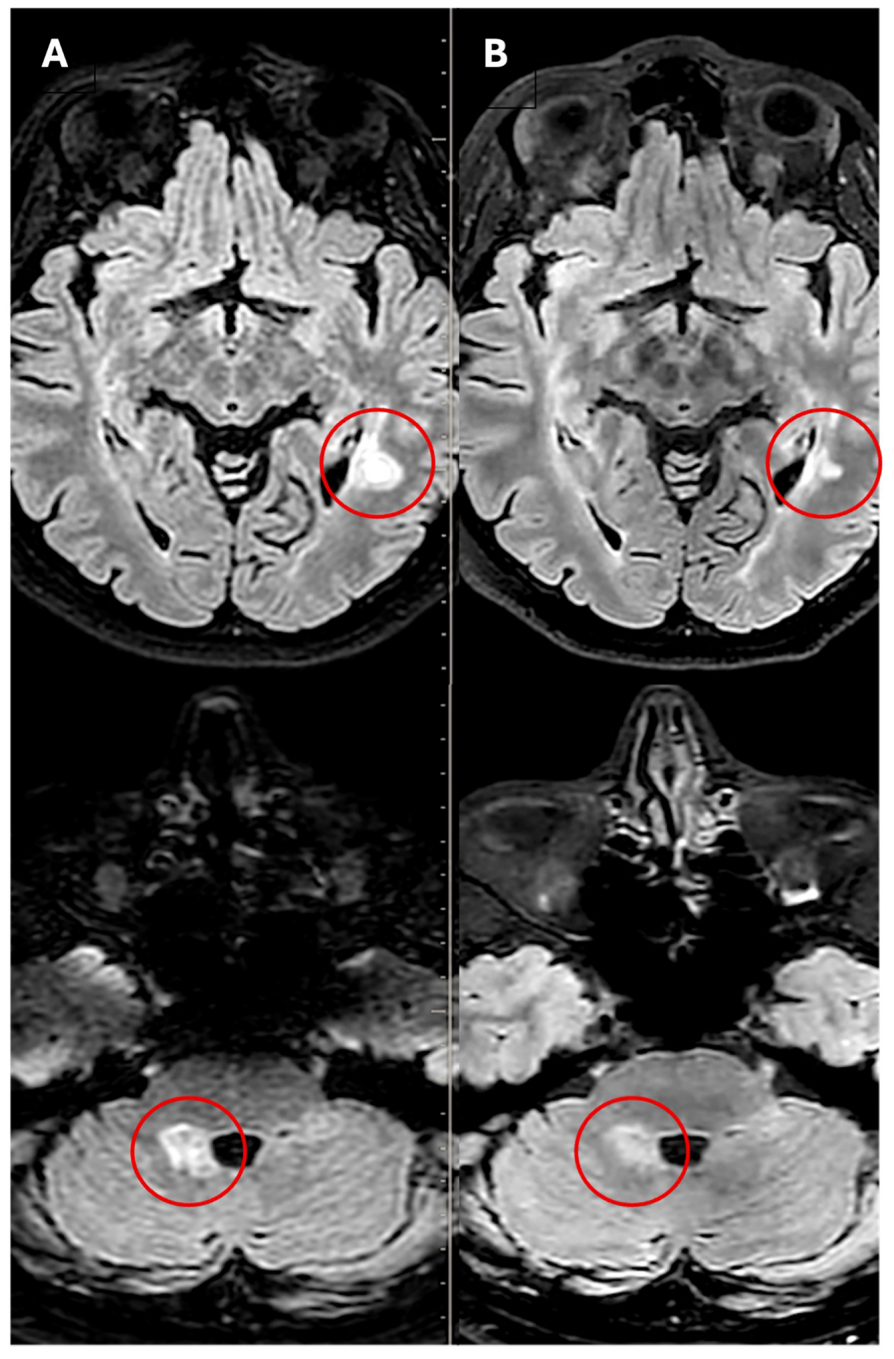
On the left (A), axial FLAIR brain MRI reveals a large demyelinating lesion extending from the globose nucleus to the middle and superior cerebellar peduncle, with partial peripheral contrast enhancement on post-gadolinium T1-weighted images (not shown). An additional lesion is seen in the left posterior temporal white matter, also showing contrast enhancement on post-gadolinium T1-weighted images (not shown). On the right (B), follow-up axial FLAIR brain MRI after Ocrelizumab therapy shows a reduction in size of both previously enhancing lesions, with resolution of contrast enhancement on post-gadolinium T1-weighted images (not shown). MRI was performed using 1.5-T and 3.0-T scanners with sequences including TSE-T2, FLAIR 3D, FFE-T2, 3D-T1, DWI, and STIR.
